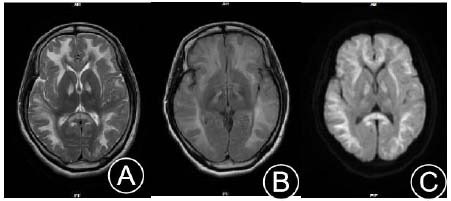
Cranial MRI: Symmetrical large patchy high signals in the basal ganglia, corticomedullary junction, centrum semiovale, and the genu and splenium of the corpus callosum on T2WI and FLAIR (A, B); DWI shows band-like high signals in the corticomedullary junction of both cerebral hemispheres (C).
